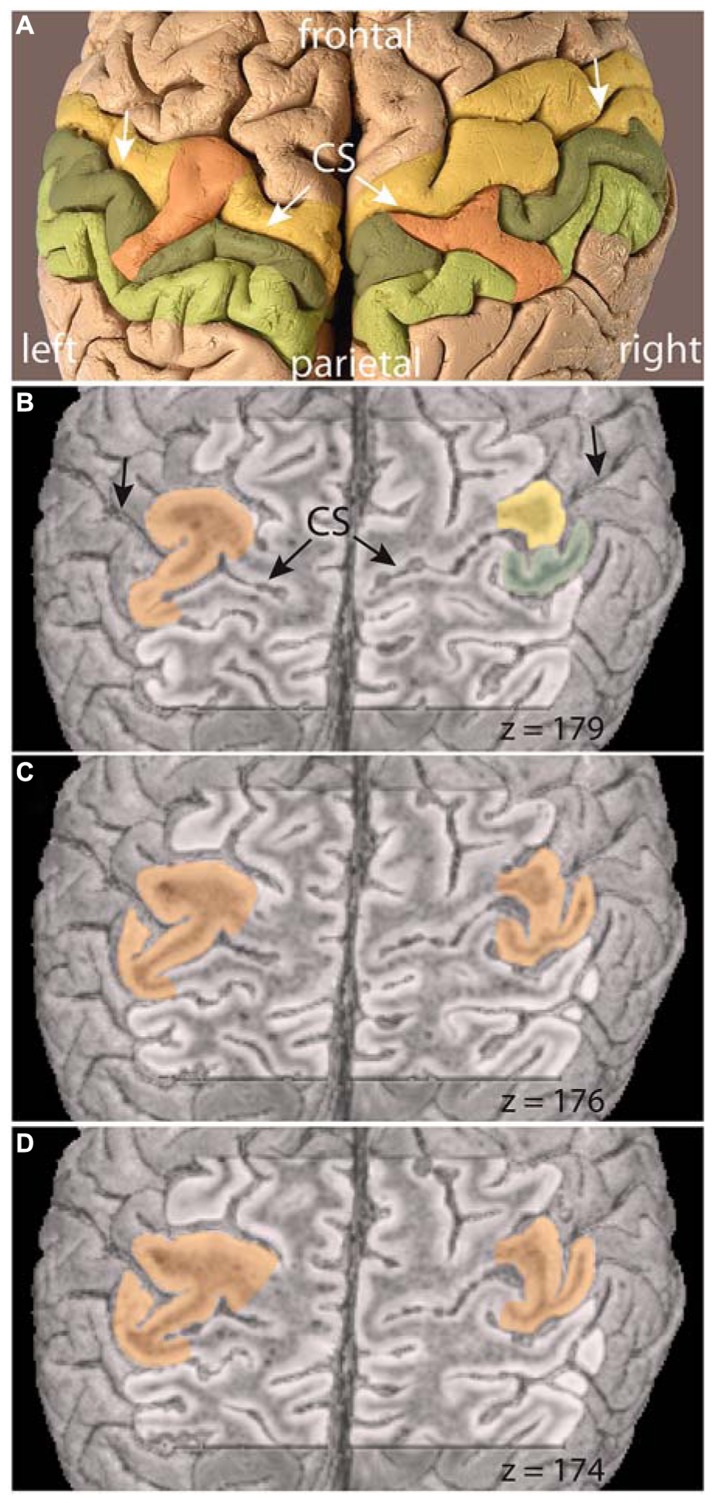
(A) Photograph and (B–D) cross-sectional MRI views of the brain of C. H. Fuchs at mid-depth level of the central sulci (CS, arrows). *z* = z-coordinate in mm, precentral gyrus = yellow, post-central gyrus = dark green, bridges = orange.
